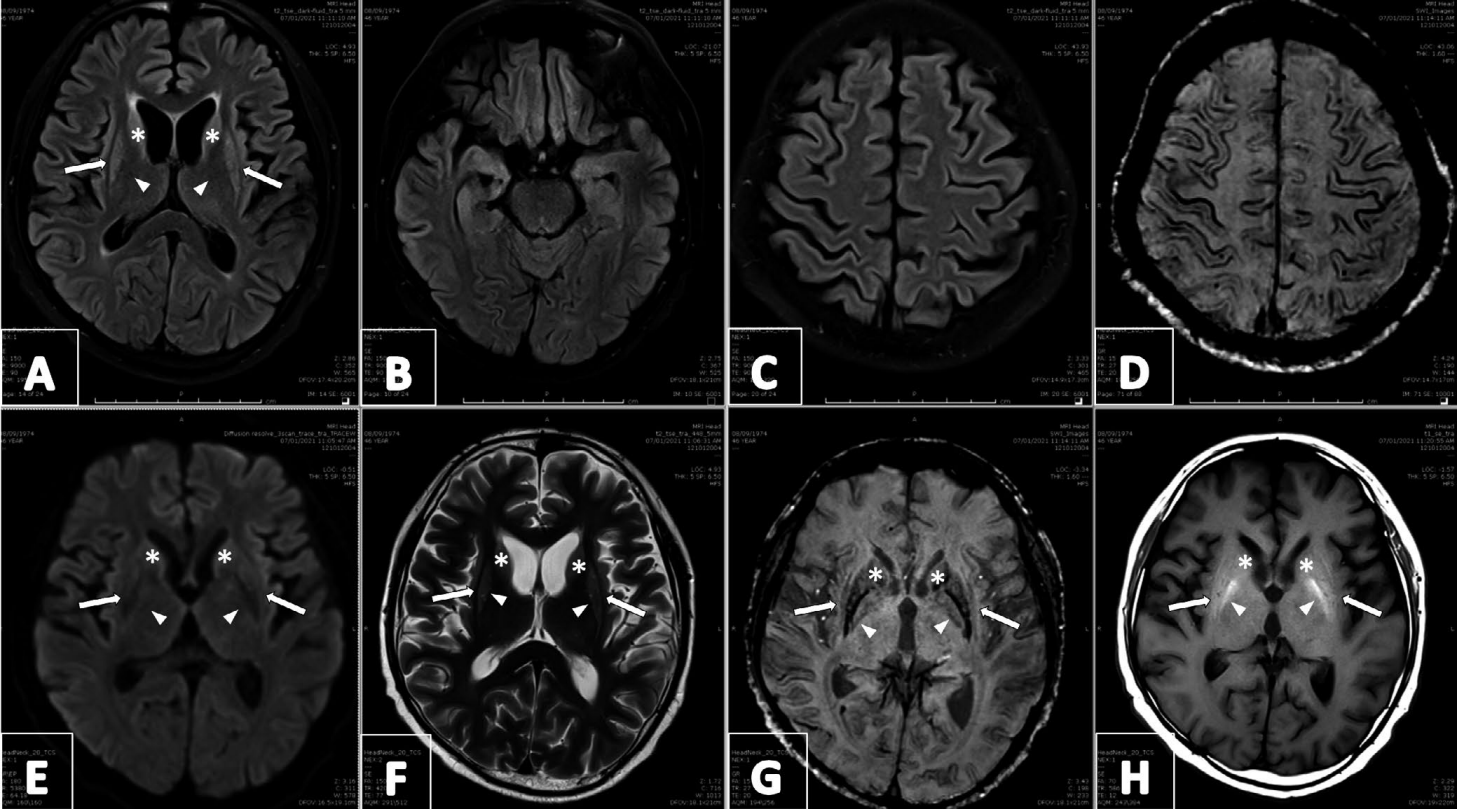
Magnetic Resonance Imaging (MRI) of the brain. (Follow‐up). Significant decrease of symmetrical swelling and abnormal signal intensity in the involved regions as compared to earlier MRI (A–F, H). SWI sequence (G) displays susceptibility changes in the parietal, subcortical, putamen, and caudate regions, suggestive of microhemorrhages due to COVID‐19‐related encephalopathy. Symbols are point out the involved regions, that is, caudate nuclei (asterisk), putamen (arrowhead), and external capsule (white arrow)
